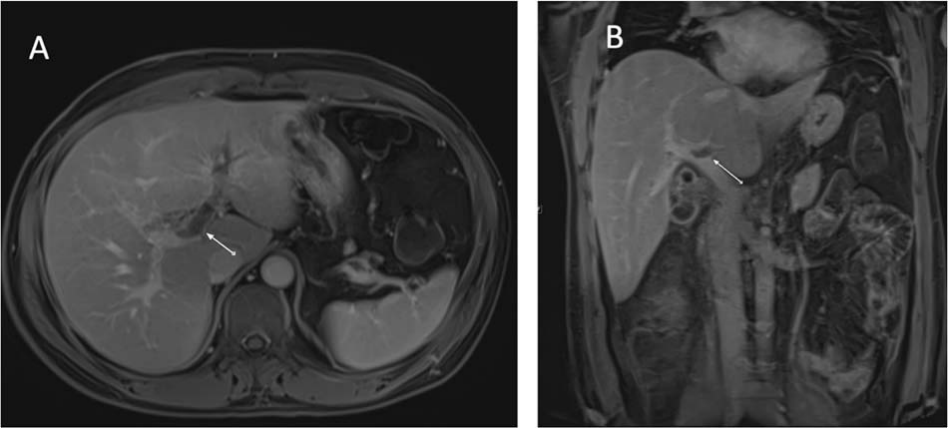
MRCP T1-weighted images: (A) axial cut; (B) coronal cut. Arrow indicates left portal vein thrombosis.
